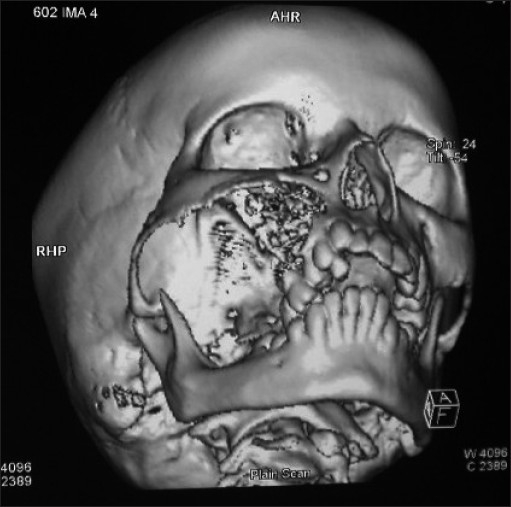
Computed tomographic image showing involvement of the maxillary sinus with the erosion of alveolar bone and medial, lateral and superior walls of the sinus
